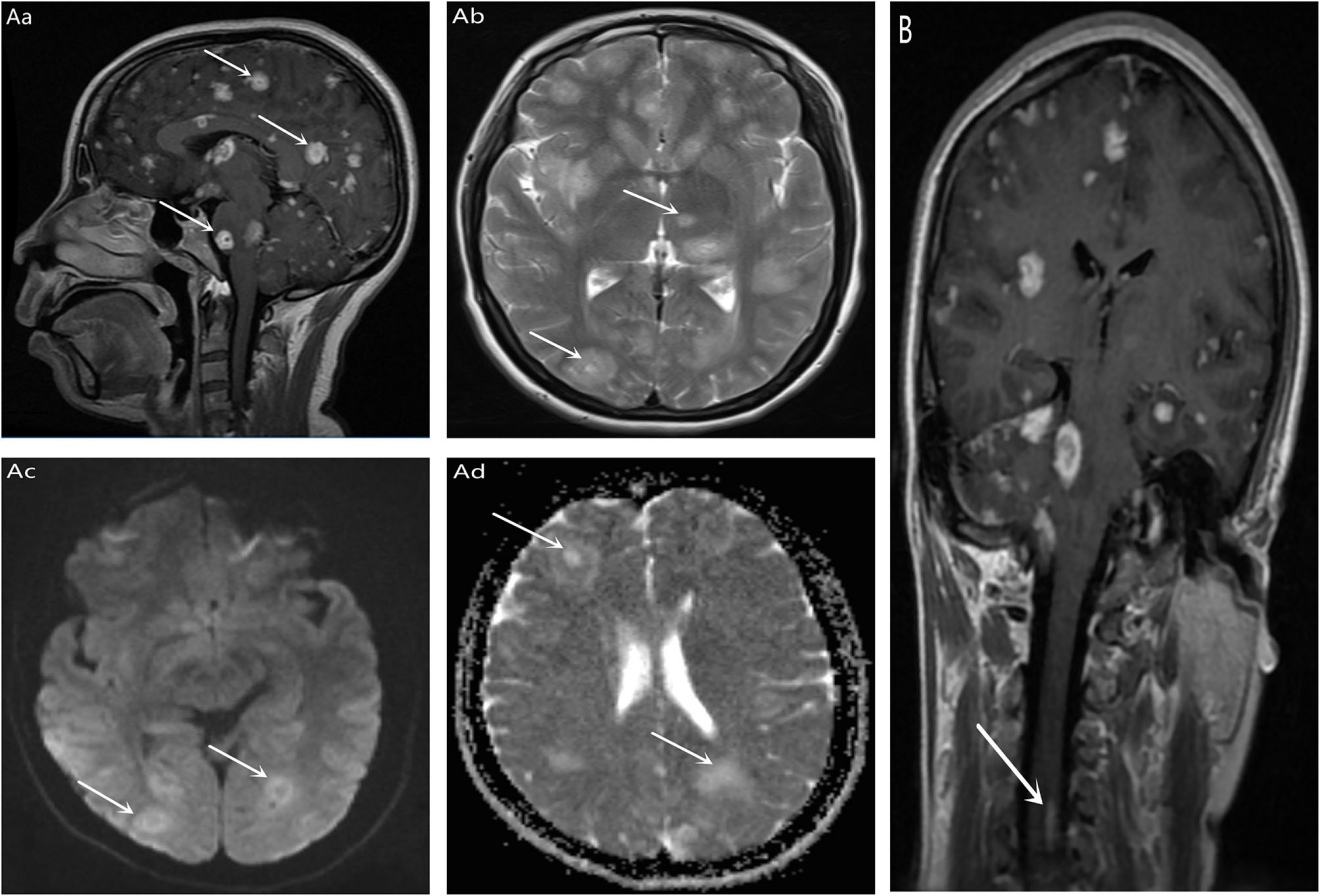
T1 (Aa) and T2 (Ab) signal of multiple Clumps long T1 in bilateral brain, cerebellum, brainstem, and corpus callosum. DWI (Ac) and ADC (Ad) showed high signal (indicated by white arrows in the figure); Enhanced scan showed bilateral nodules, cerebellum and brainstem multiple nodular, annular enhancement, the largest diameter of about 18 mm (indicated by the white arrow in the figure). (B) No separate MRI of the spinal cord was performed, and there was also an unexpected high signal in the spinal cord during cranial enhancement (indicated by the white arrow in the figure).
